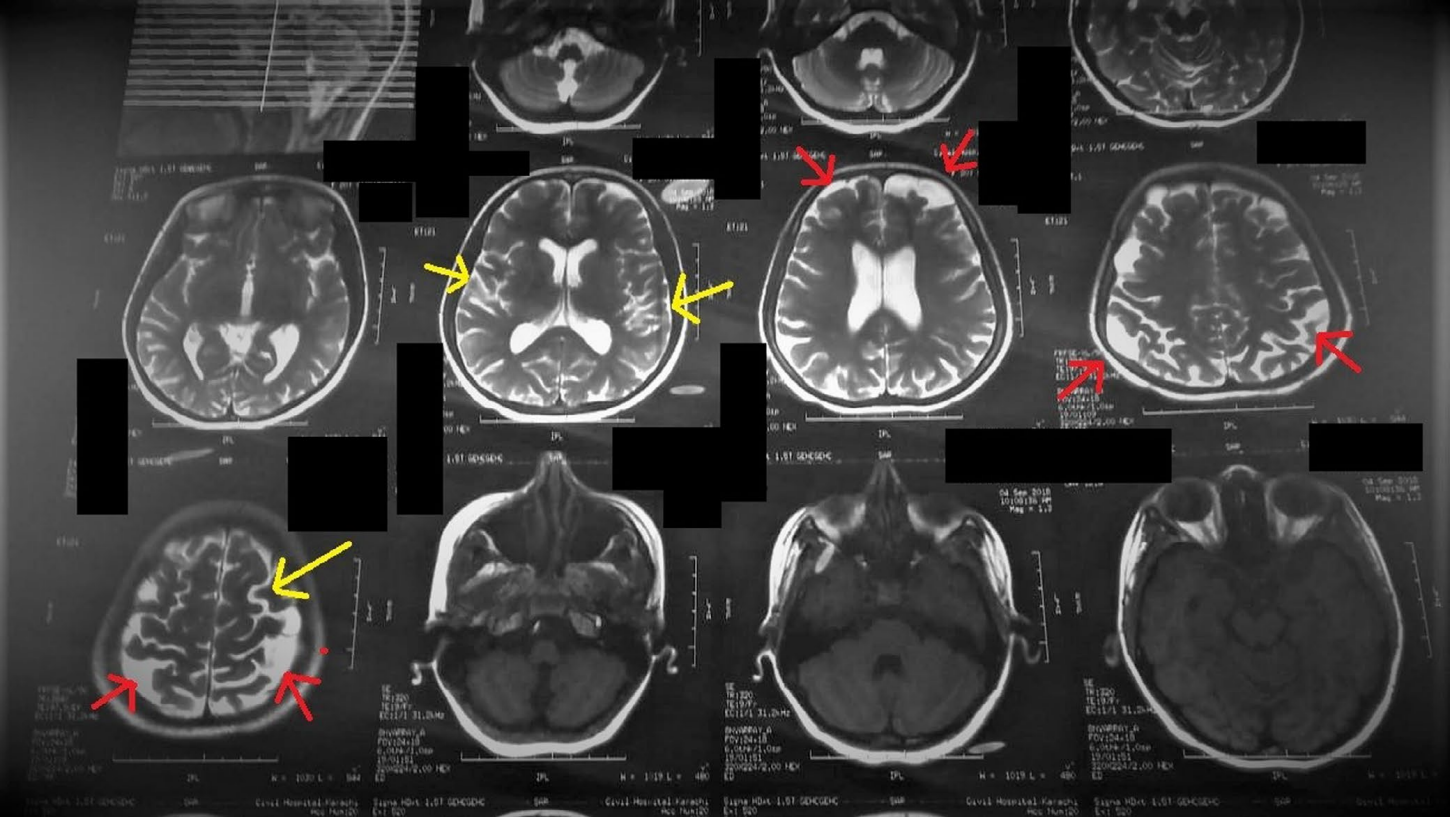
MRI of the brain shows multiple cerebral atrophy with an expansion of sulci, extra‐axial spaces, and thinning of gyri (yellow). Bilateral multifocal T2 and FLAIR hyperintense signals were seen in subcortical regions of fronto‐parietal lobes bilaterally (red)
